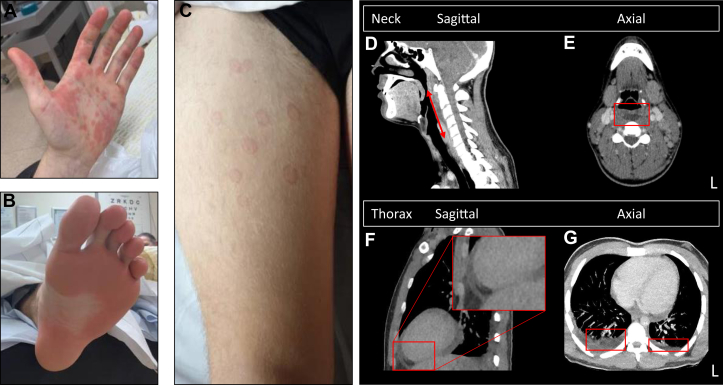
A, Multiple sharply demarcated erythematous papules and plaques on the palmar skin. B, Diffuse erythema of the plantar skin. C, Multiple annular erythematous scaling patches on the proximal extremity. D and E, Sagittal CT scan (D) and axial CT scan (E) of the neck, showing rim-enhanced retropharyngeal fluid collection, 71 mm caudocranial to 11 mm anteroposterior. F, Sagittal CT scan of the chest, showing inferolateral pericardial effusion with a maximum thickness of 12 mm. G, Axial CT scan, showing subtle bilateral pleural effusion.
